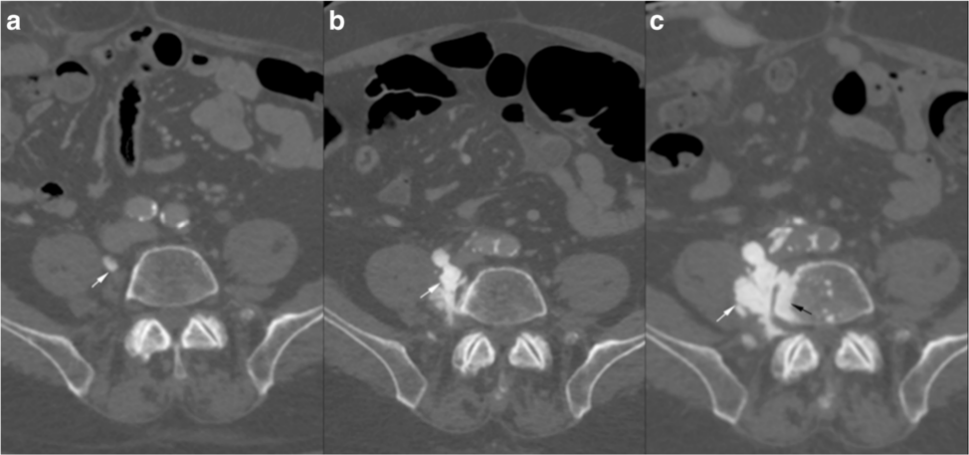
Follow-up CT performed 1 year after surgery (a) shows a small calcified nodule (white arrow) in the right retroperitoneal space. 6 months later (b) the calcified mass has increased in size. On another control 6 (c) months later the ossification progressed in size and into the adjacent vertebra (black arrow)
